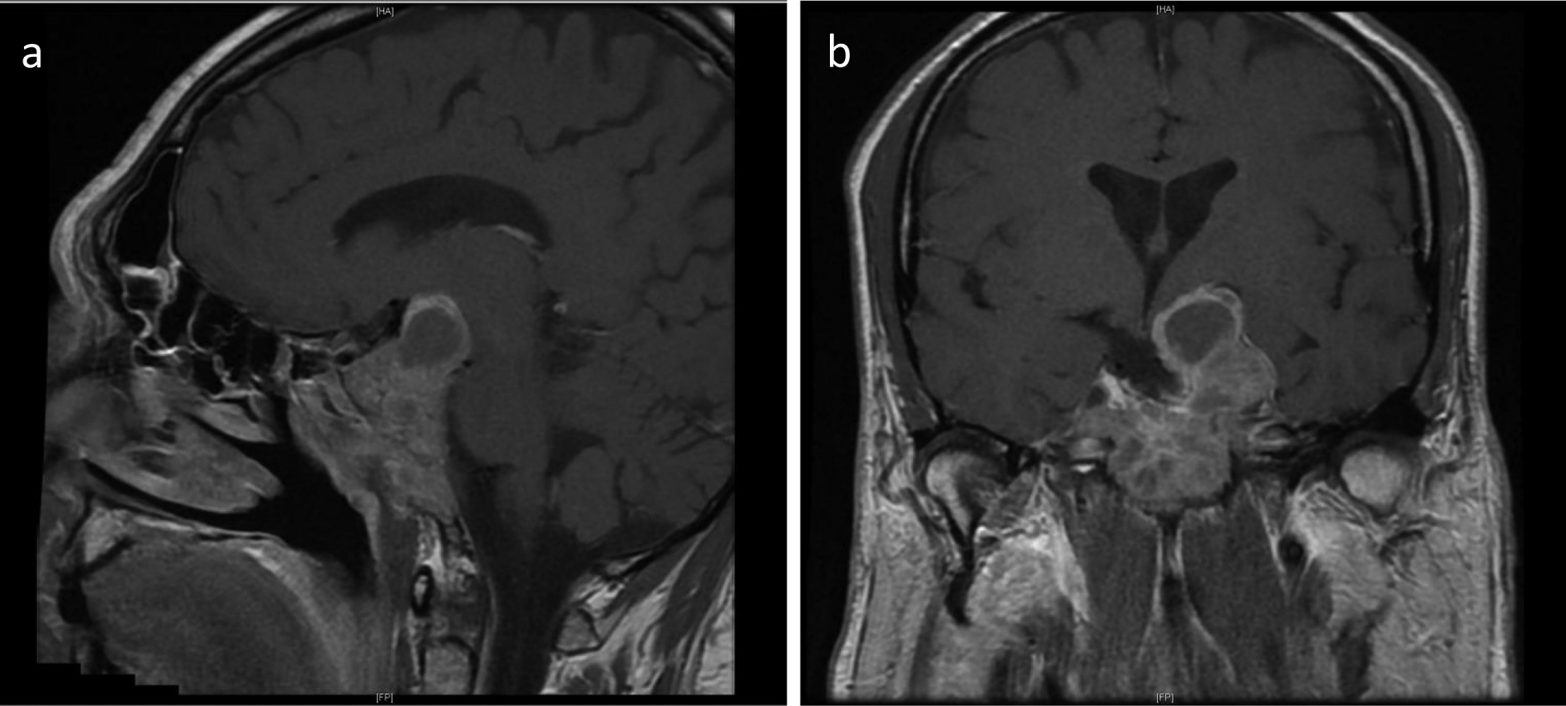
(a) Postoperative *T*_1_ weighted sagittal and (b) coronal MRI scan with contrast demonstrated extensive residual disease at 4 months following decompression surgery. Maximum tumour dimensions were 61, 50 and 34 mm in the craniocaudal, transverse, and anteroposterior planes, respectively.
